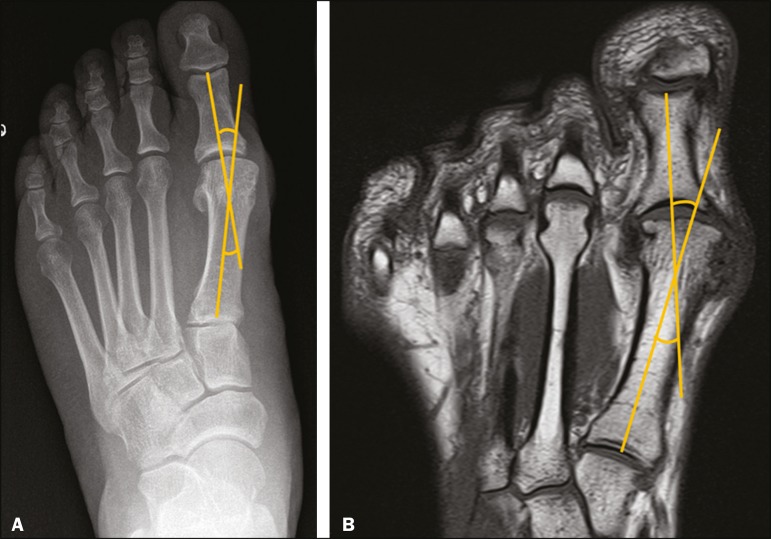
Technique for measurement of the HVA on plain radiography (A) and in a T1-weighted MRI sequence (B). The HVA was measured on MRI in a manner analogous to that recommended for plain radiography, based on two lines crossing the long axis of the diaphysis of the first metatarsal bone and the proximal phalanx of the hallux.
